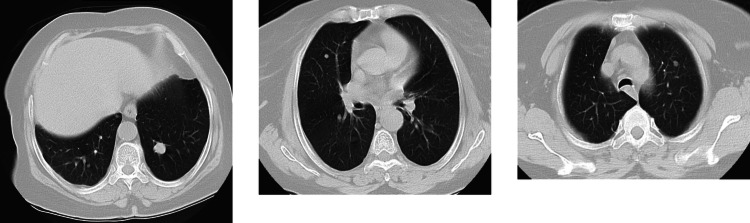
Sequential thoracic CT scans showing pulmonary metastases and treatment response. (A) Baseline CT (June 2023): multiple bilateral pulmonary metastases. (B) After 3 months of pembrolizumab (October 2023): partial reduction in lesion size. (C) After 6 months of treatment (March 2024): complete radiologic remission of lung lesions
